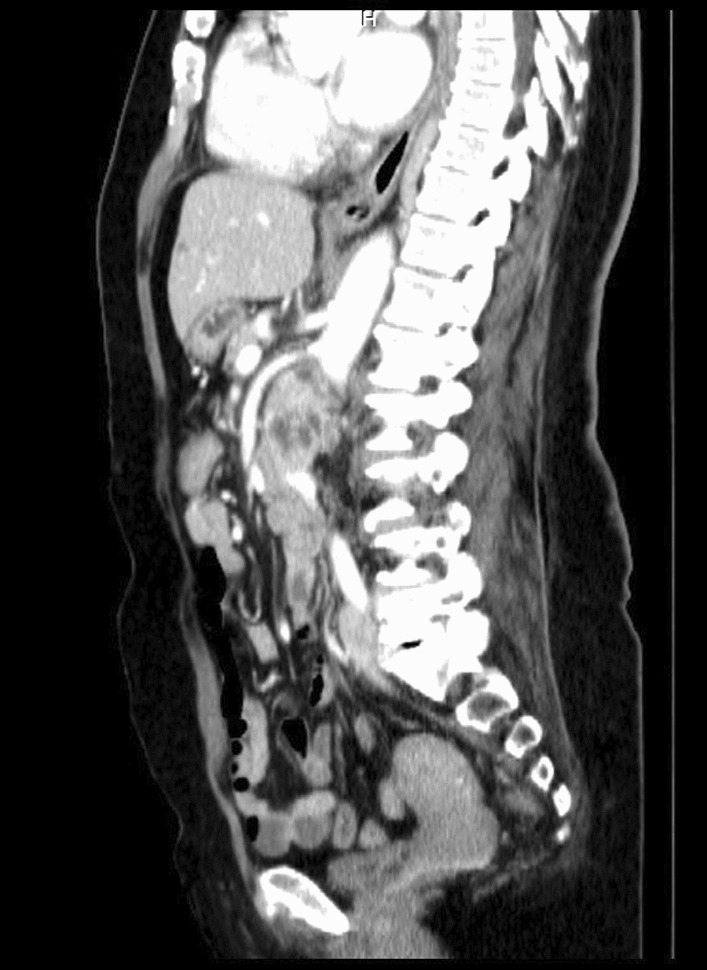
Preoperative computed tomography angiography, sagittal view
